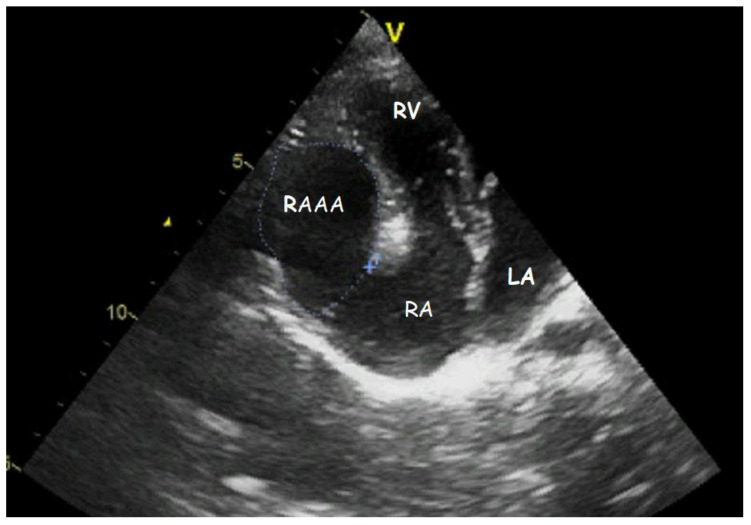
Transthoracic echocardiogram. Modified four−chamber view showing the gigantic RAAA.
